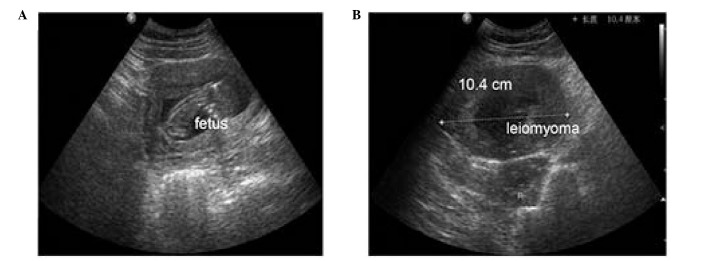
B-mode ultrasound showing (A) the fetus and (B) a right, hypoechoic adnexal mass.
